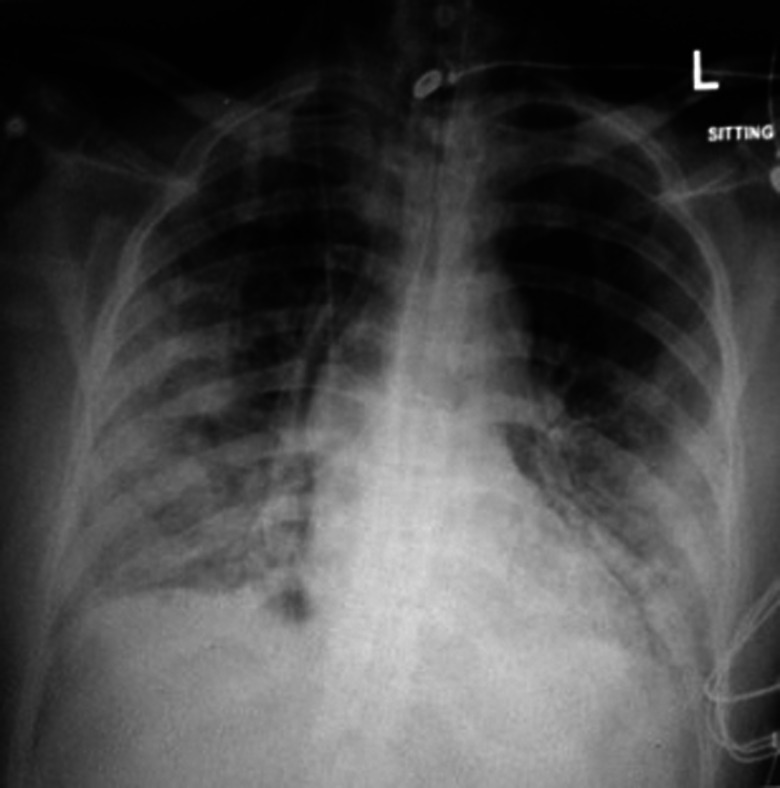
Chest x-ray showing spontaneous pneumomediastinum.
